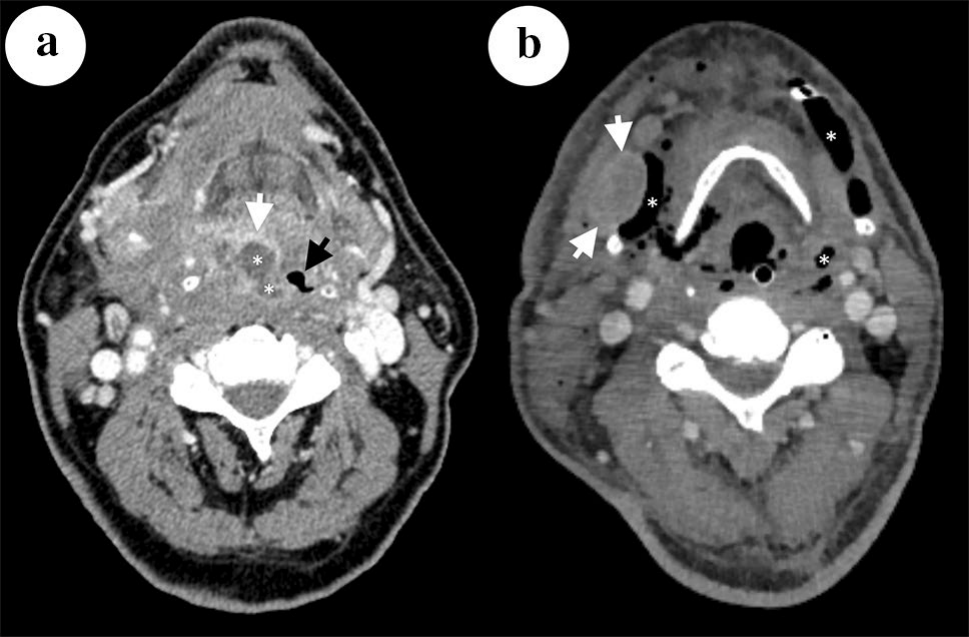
a Case of phlegmon of parapharyngeal space arising from right palatine tonsil in 74-year-old Caucasian male on axial CT image with contrast enhancement. Low attenuation fluid area (*asterisk*) with rim of enhancement (*white arrow*) and surrounding soft-tissue edema in right parapharyngeal space cause deviation of the pharynx and narrows its lumen (*black arrow*). b Case of phlegmon with gas formation arising from right palatine tonsil in 64-year-old Caucasian male on axial CT with contrast enhancement. Gas is visible between soft tissues (*asterisk*). Enlarged and heterogeneously enhancing lymph node in right submandibular region is present (*arrows*). In addition, subcutaneous tissue edema manifests as fat stranding
